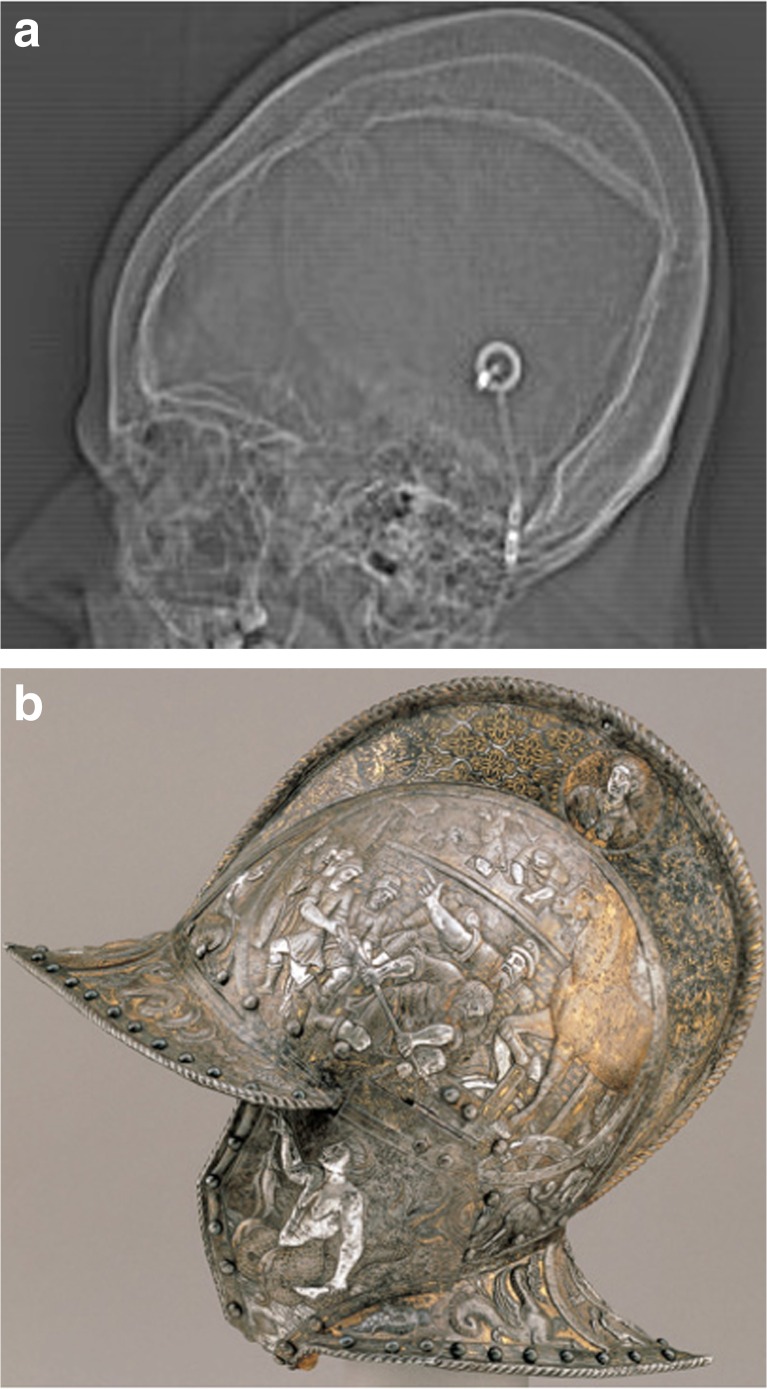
Toposcan CT: double calcification of the skull (a). Note the similarity with an armored helmet (b)
